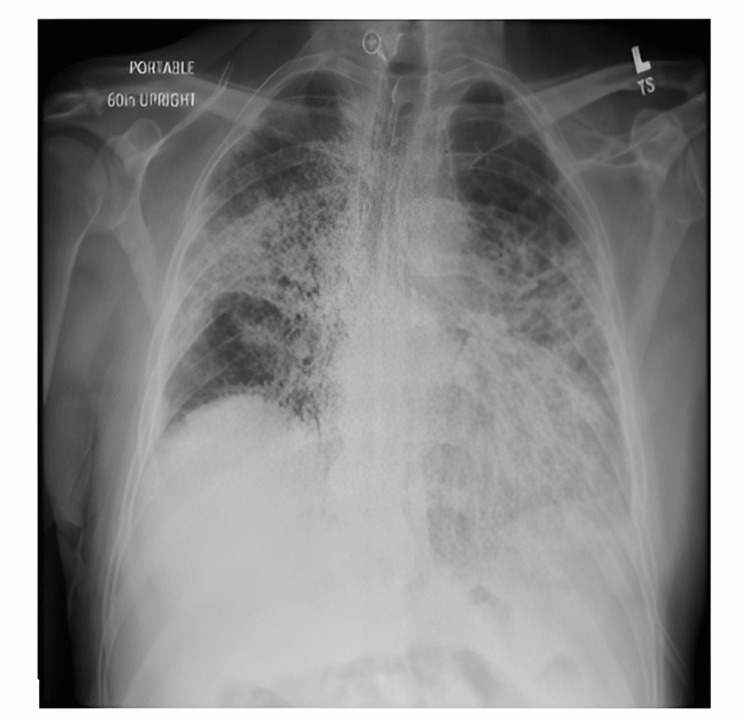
Antero-posterior view of chest X-ray demonstrating multifocal pneumonia
